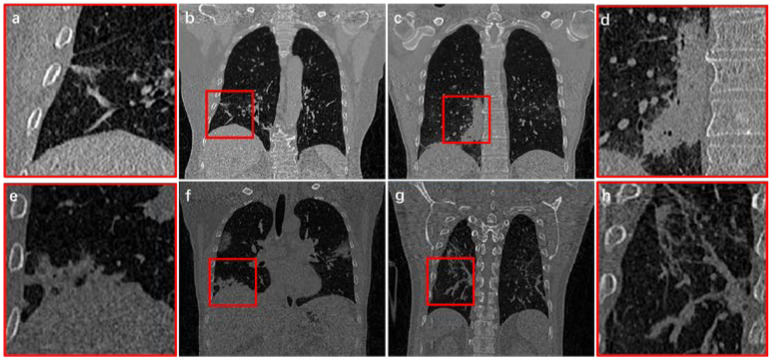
Infection structure from COVID-19 CT images. (b,c,f,g) indicate four different cases. (a,d,e,h) are the local magnification structure of the red boxes from (b,c,f,g), respectively.
